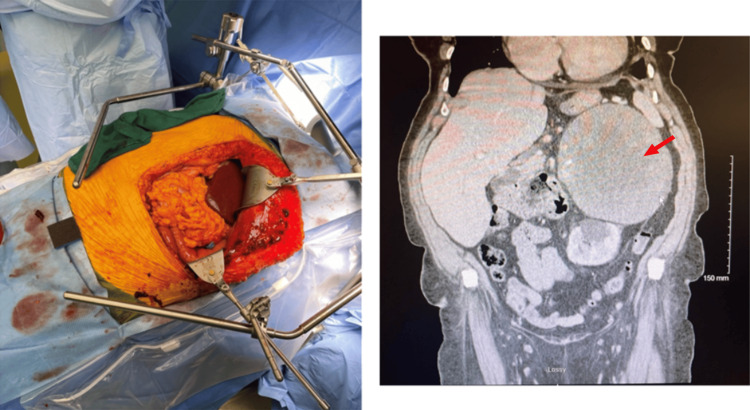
Intraoperative view of left-sided modified Makuchi incision (left); patient's CT scan showing a 13.9 cm left adrenal mass (right).
